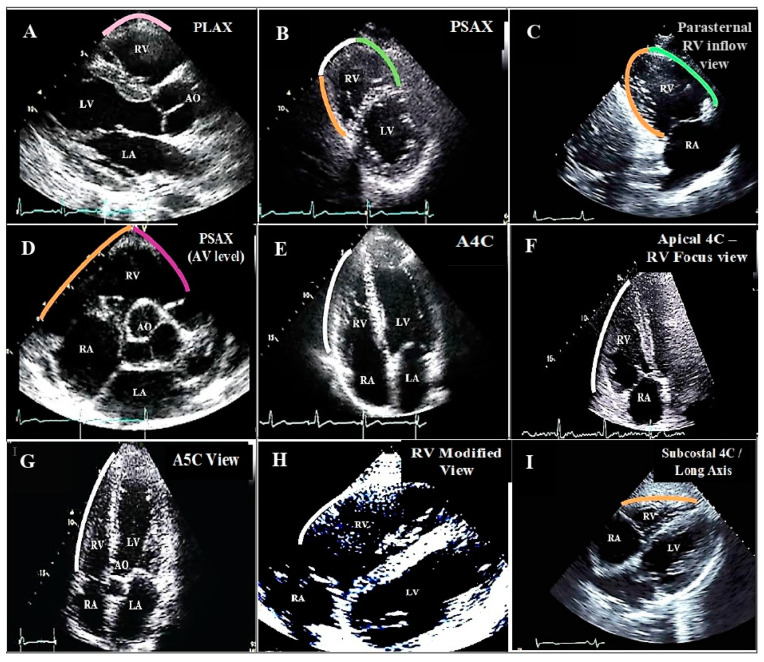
Different transthoracic and subcostal echocardiographic views displaying different right ventricular regions.
